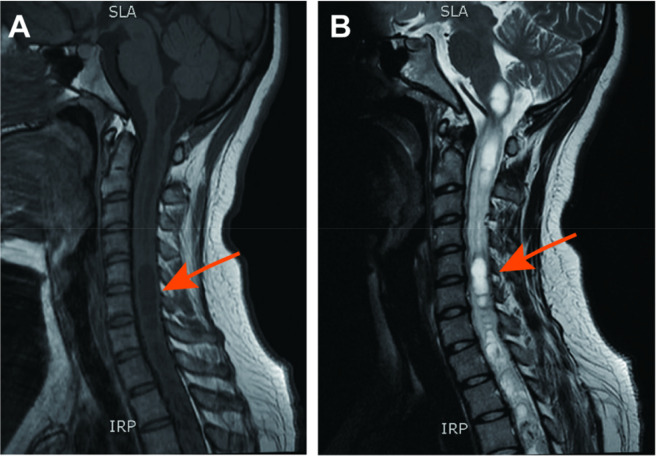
Spinal cord magnetic resonance imaging in 2020 showing a space-occupying lesion within the spinal canal at the T2-T5 level. (A) T1-weighted magnetic resonance image. (B) T2-weighted magnetic resonance image.
